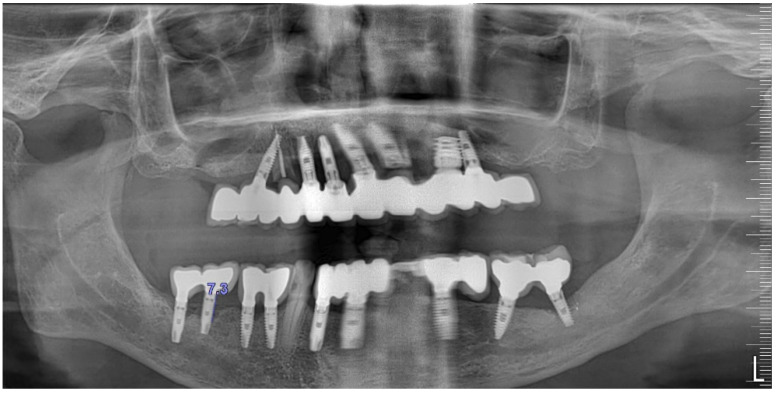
The initial panoramic radiograph reveals oral rehabilitation and bone loss in the lower posterior teeth.
